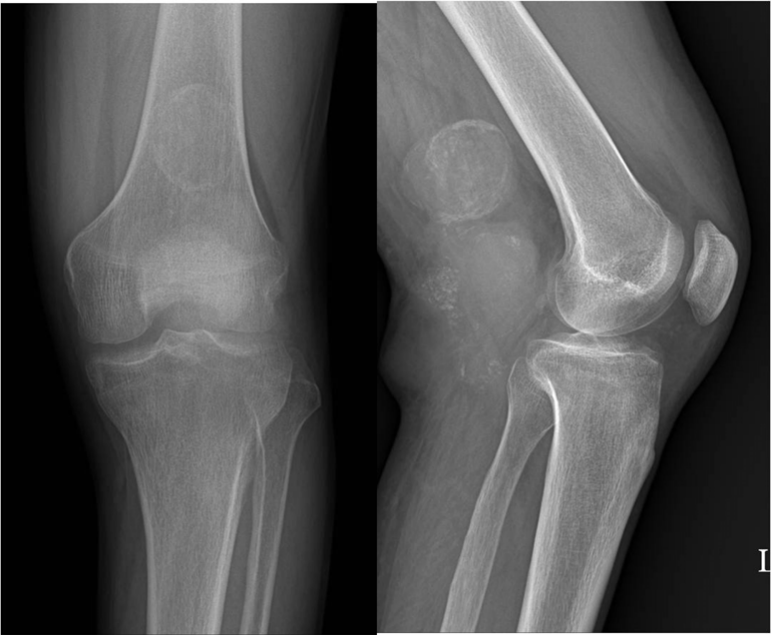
A plain radiograph showing a well-defined soft tissue mass with marginal calcification posterior to the distal femur. There is no evidence of associated bone erosion in this patient. This is an example of a synovial sarcoma in one of its more common locations.
